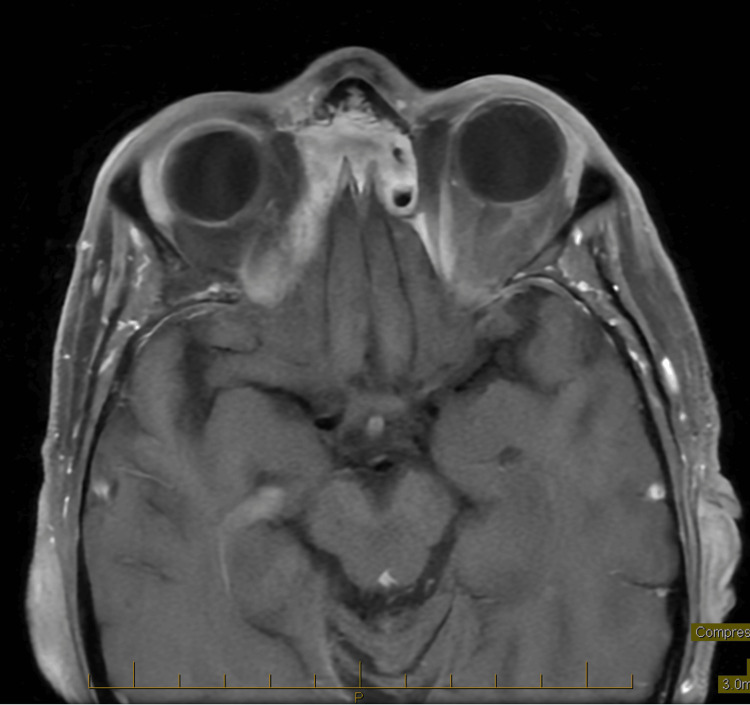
Post-chemotherapy MRI orbits T1+c (July 9, 2024). MRI: magnetic resonance imaging
